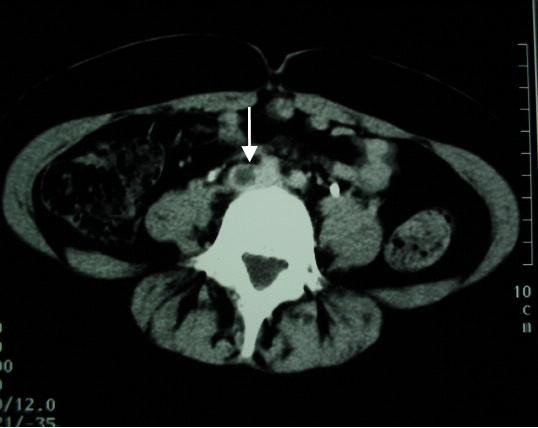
Abdominal computed tomographic scan showing a common iliac vein thrombosis.
